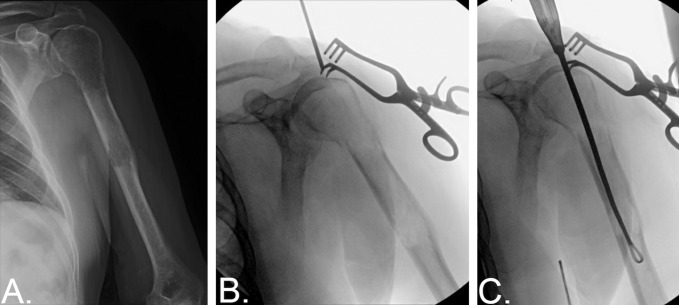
A, Radiograph of the humerus demonstrating lytic lesions of both the humeral shaft and proximal humerus with notable cortical compromise, resulting in nondisplaced pathologic fracture of the surgical neck. B, Intraoperative fluoroscopy demonstrating identification of the starting point for instrumenting the humerus. C, Intraoperative fluoroscopy depicting the use of a looped curet through the starting point in the humeral head to grossly debulk the tumor.
